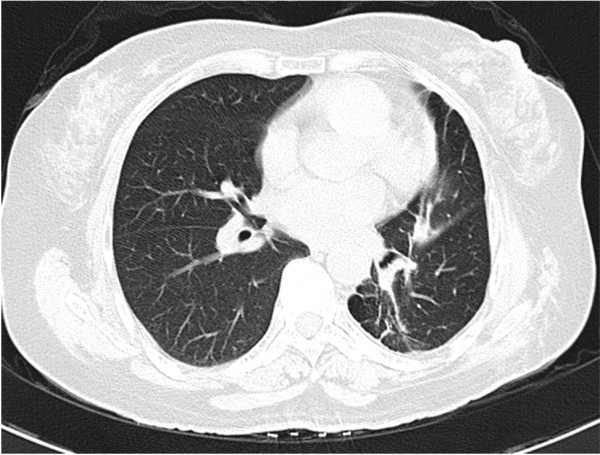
Computed tomography scan of Case 1 obtained in 2011. Computed tomography scan obtained in 2011 indicating good expansion of the left lung and a fibrous lesion in the area on which surgery was performed.
